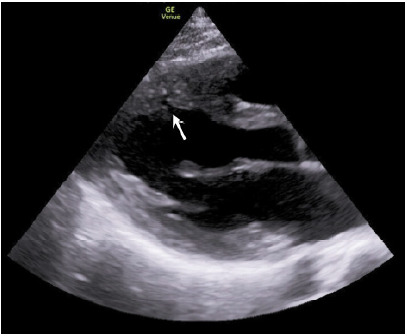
Point-of-care ultrasound echocardiogram on parasternal long-axis view demonstrating an invagination within the anteroseptal wall of the interventricular septum, known as a myocardial crypt (arrow). No septal hypertrophy, left ventricular outflow tract obstruction, or other structural or kinetic abnormalities were appreciated.
